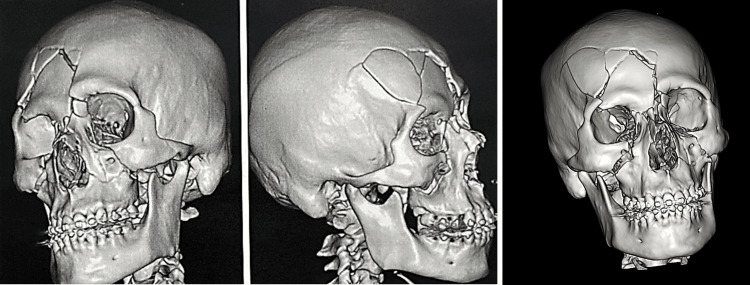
Three-dimensional CT reconstruction of the skull and facial skeleton Left and center: lateral views showing a multifragmented right frontoparietal fracture with depression and orbital involvement. Right: oblique view demonstrating extension to the orbit (roof, lateral wall, and floor), a Le Fort II fracture, and a right NOE type III injury. CT: computed tomography; NOE: naso-orbito-ethmoidal
